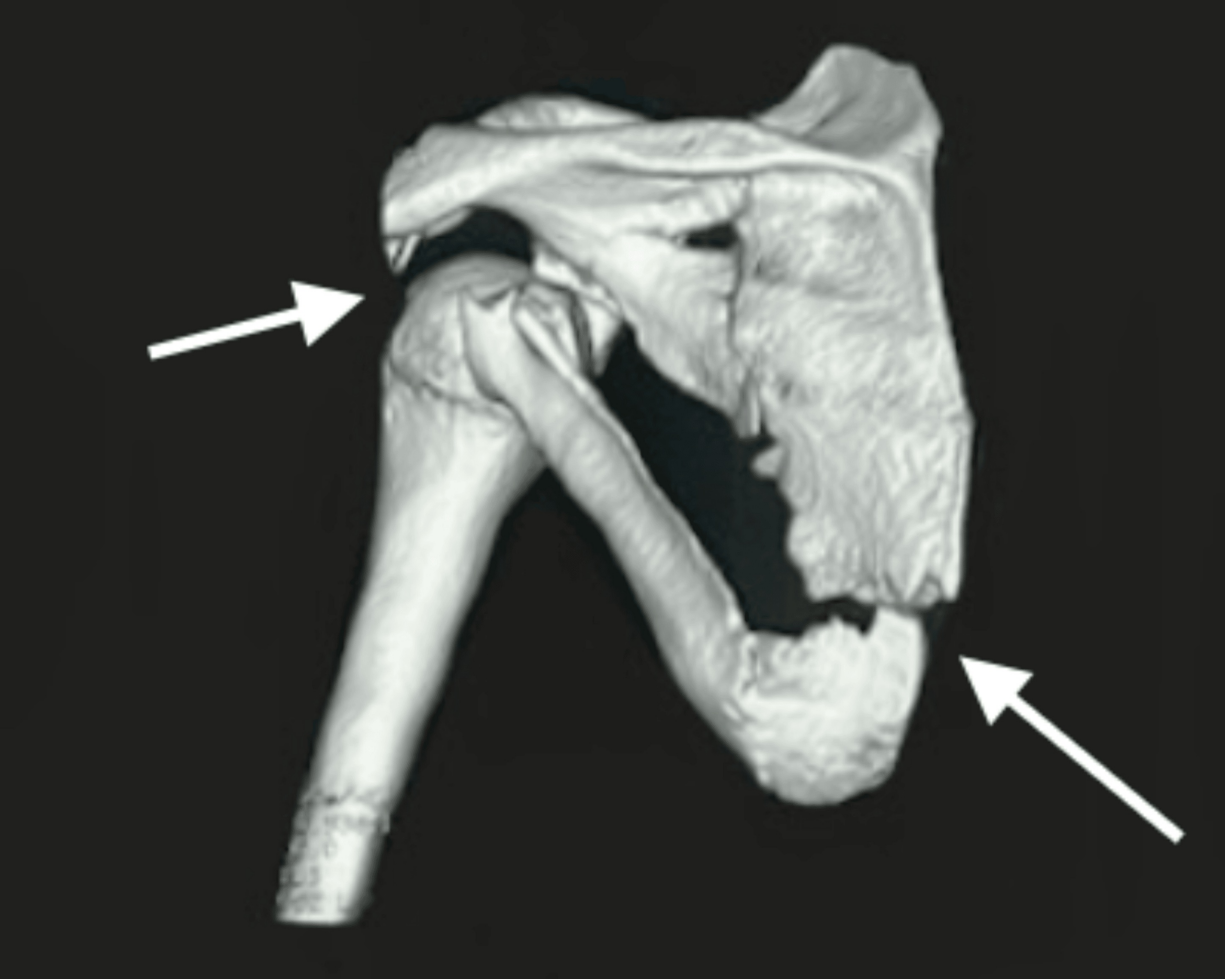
Left shoulder CT bone reconstruction with evidence of multifragmentary fracture involving both the scapular body and the glenoid cavity. CT: computed tomography
